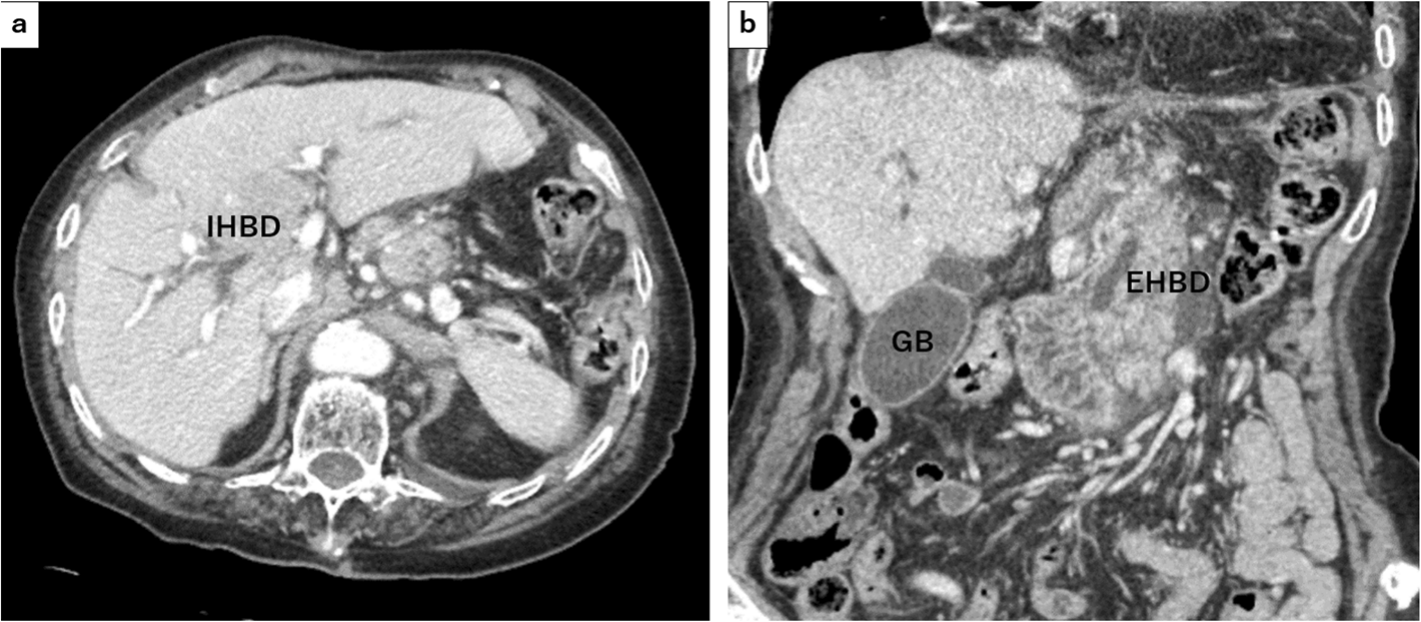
Enhanced computed tomography (eCT) revealed that the intrahepatic and extrahepatic bile ducts were dilated, and the latter was bended. In axial view (a) and in coronal view (b). IHDB: intrahepatic bile duct, EHBD: extrahepatic bile duct, GB: gallbladder
